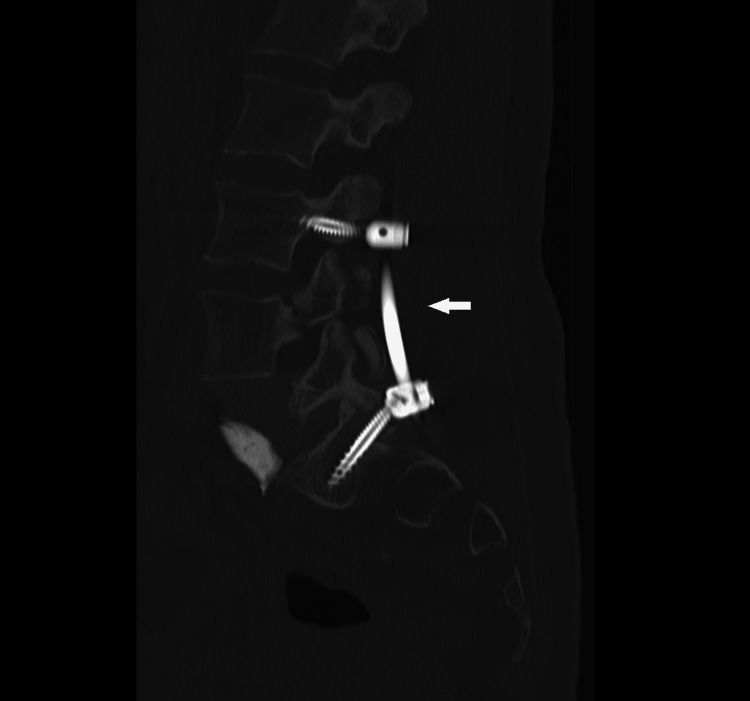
Lumbar spine CT scan after surgical posterior stabilization L3-S1.
